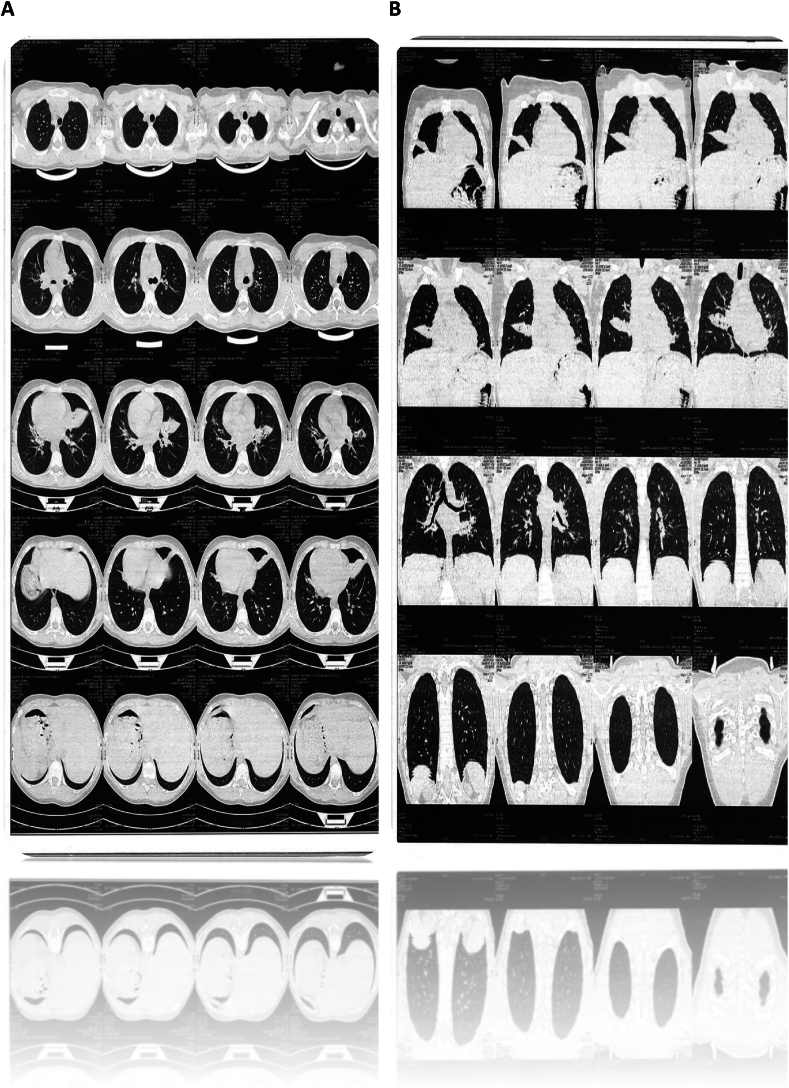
Axial CT scan of the chest plain Axial CT Scan Display a Series of Cross-Sectional Images. In the review of the CT axial Chest CT scan, it reveals the presence of patchy opacities seen in the right middle lobe & band like opacity with mild bronchiectatic changes seen in the lingula Segments of left upper lobe. The opacities were distributed in non-uniform pattern across the multiple CT images.
